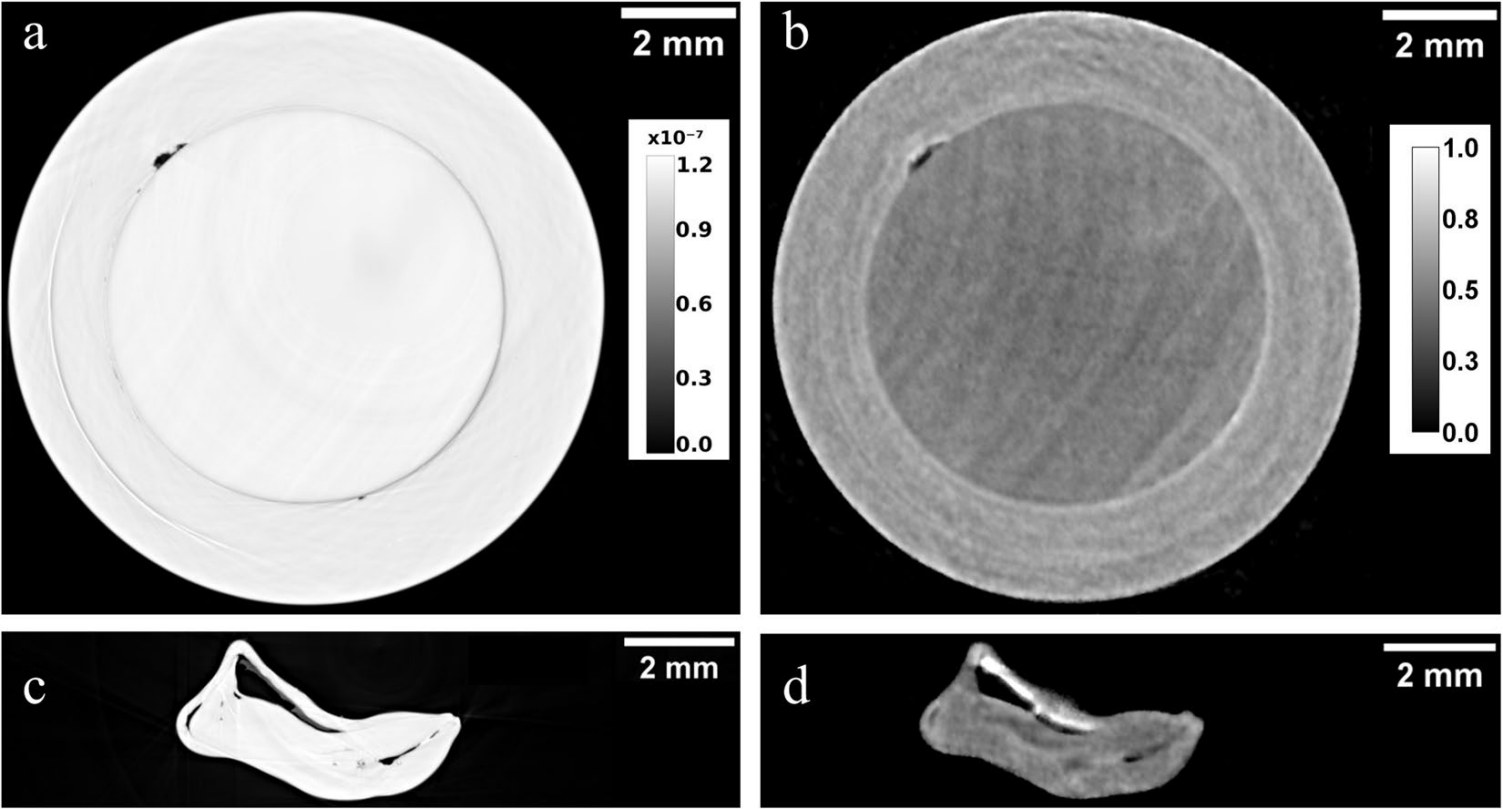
X-ray PC (a) and neutron (b) CT reconstructed images corresponding to a slice placed in the middle of the main part of the pearl CP-17. X-ray PC (c) and neutron (d) reconstructed images corresponding to a slice cutting the small attached pearl of CP-17. Organic tissue within the small attached pearl is recognized as grey zones in the neutron image (d) and white in the X-ray PC image (c). Grey scale refers to refractive index decrement for PCI (a) and to linear attenuation coefficient (cm^−1^) for NI (b).
